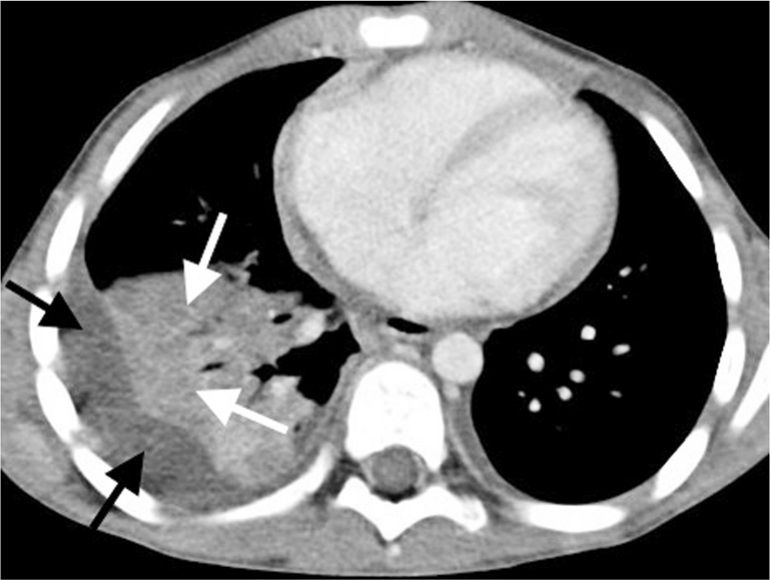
Contrast-enhanced axial CT of the chest in a 5-year-old boy demonstrates an effusion (*black arrows*) associated with an air-space process. The patchy low-density areas with less enhancement in the consolidated lung are in keeping with necrotising pneumonia (*white arrows*) and the patient required prolonged antibiotic treatment
